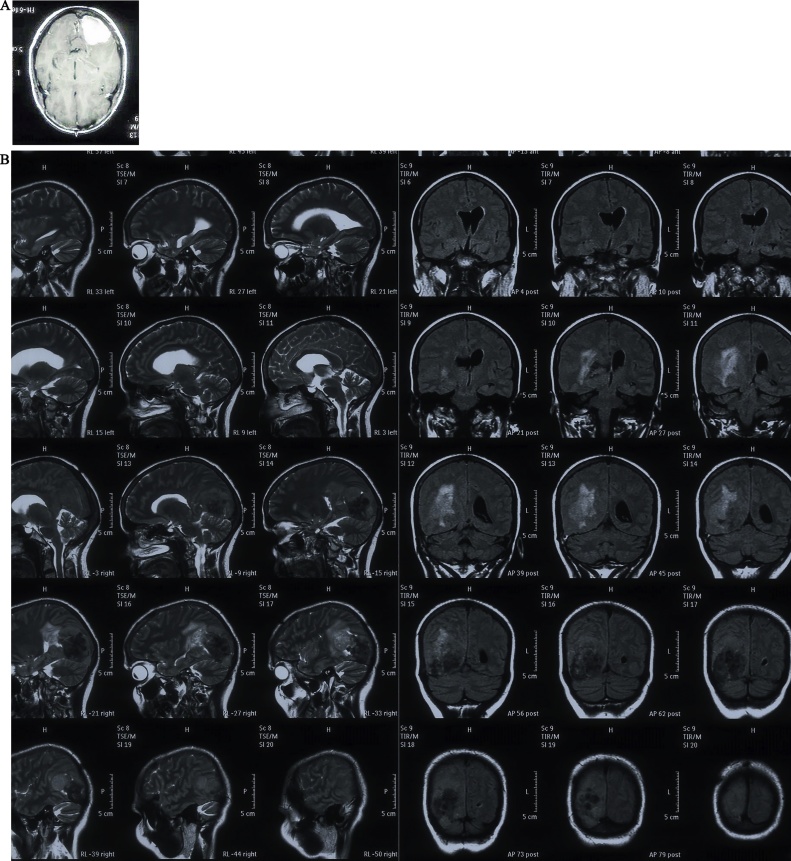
A: On MRI an ovoid mass lesion seen in the right parieto-occipital region measuring 10 × 7 × 4 cm with peritumoral edema. B: The tumor showed intermediate-low signal intensity in the T1-Weighted Image (T1WI). The mass was attached to tentorium and was seen extending into the right transverse sinus.
